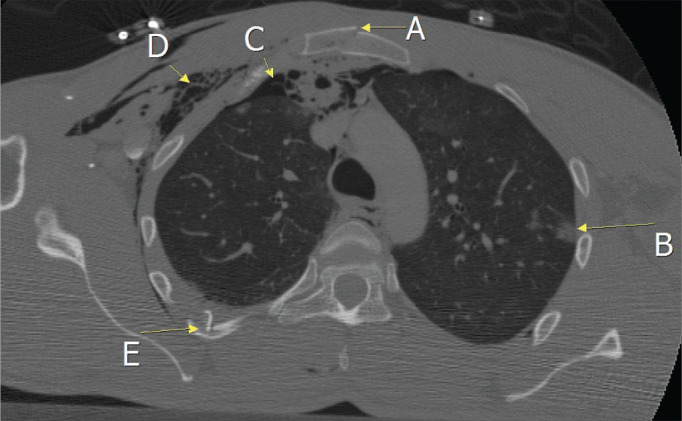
CT scan of a patient admitted because of a traffic accident, showing a
